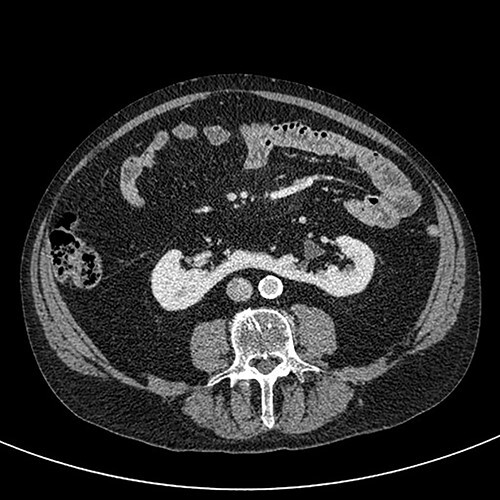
Axial CT (nephrographic phase).
